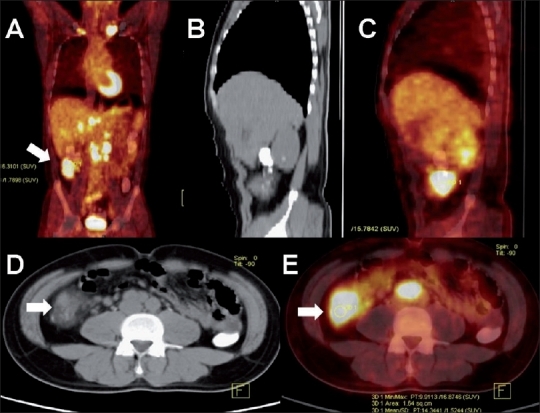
Tuberculosis of the cecum. Coronal PET/CT (A), sagittal plain CT reconstruction (B), sagittal PET/CT (C), axial plain CT (D), and axial PET/CT (E) images of the abdomen in a 36-year-old man with generalized lymphadenopathy shows increased FDG uptake in the cecum (arrows), with irregularly thickened walls (SUV 16.8) and multiple enlarged supraclavicular and abdominal lymph nodes. Disseminated carcinoma of the cecum was suspected. Colonoscopic biopsy of the cecal lesion and cervical lymph node biopsy, both revealed tuberculosis. The patient responded well to antituberculous therapy
